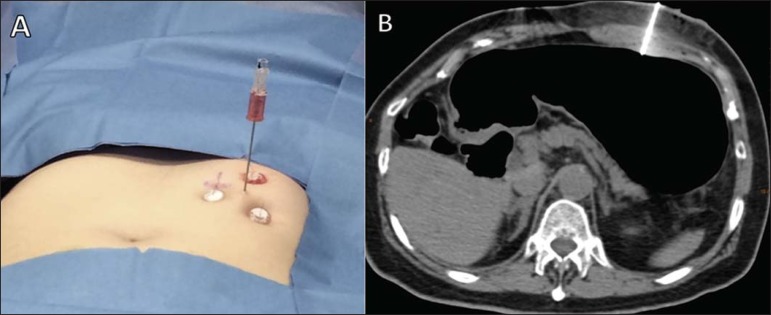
Gastric puncture for CT-guided percutaneous gastrostomy. A
